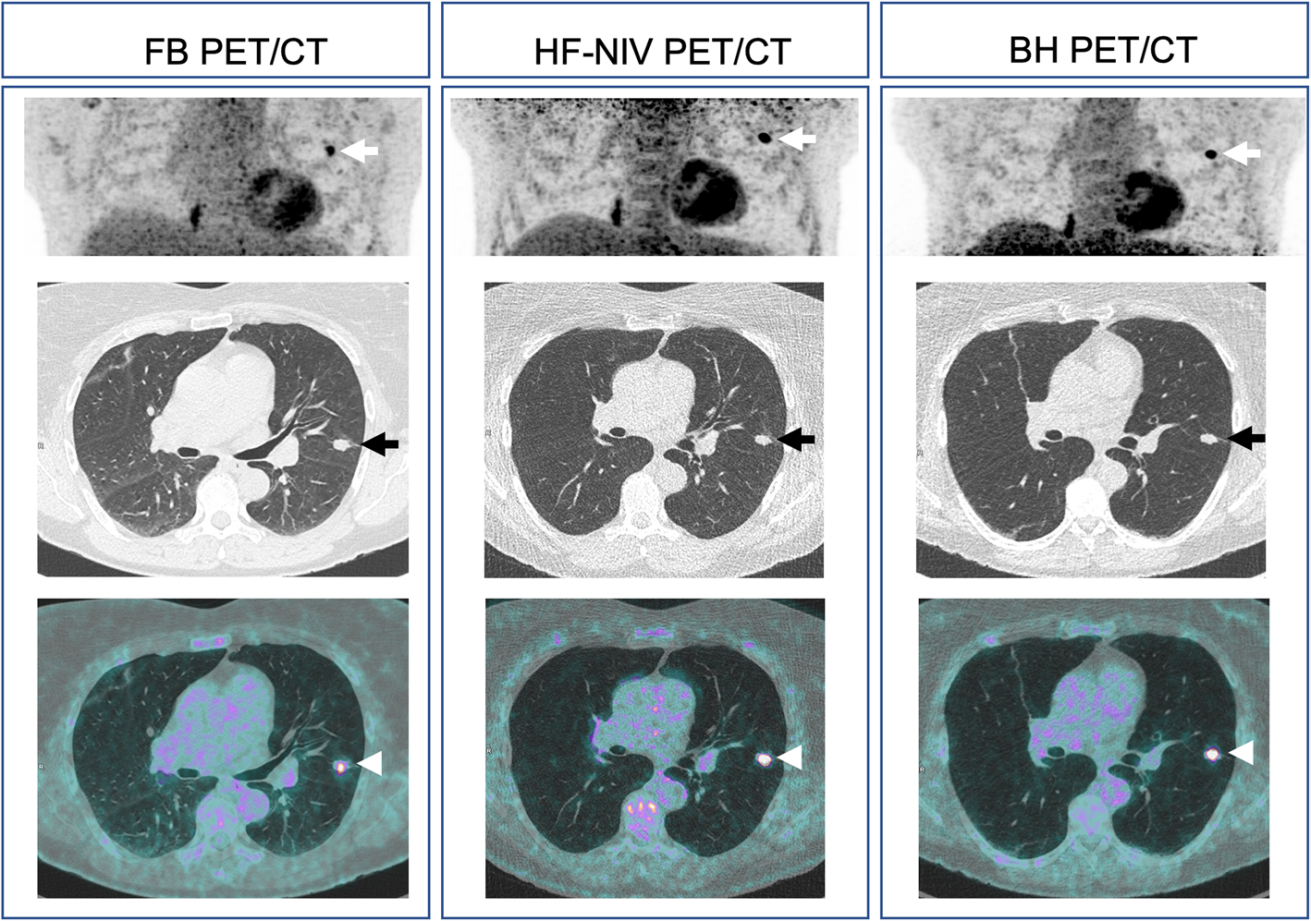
Example. A 60-year-old female patient with a history of splenic marginal zone lymphoma underwent PET/CT for characterization of a left lower lobe pulmonary nodule, shown on PET maximal intensity projection (white arrows), CT images with lung kernel (black arrows) and fusion images (arrow heads). The measured nodule long axis (mm), short axis (mm) and volume (mm^3^) were 17, 9 and 1003 on FB, 16, 9 and 802 on HF-NIV and 15, 7 and 793 on BH
